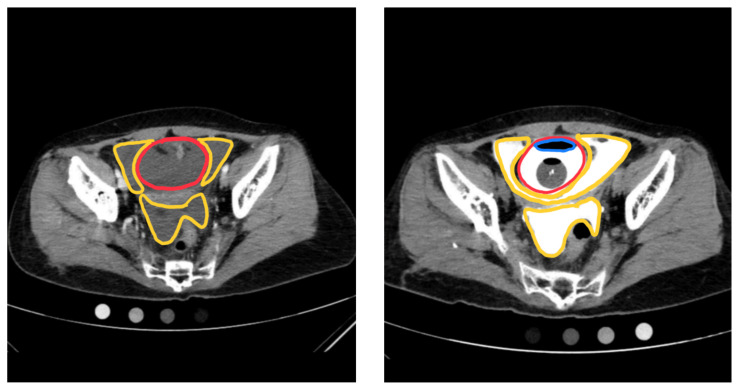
Annotated CT Video Link: https://youtu.be/1nrVhipzC08Unannotated CT Video Link: https://youtu.be/6kE88u7I55ACT Cystography Video Link: https://youtu.be/_uNbLBdYOkE
